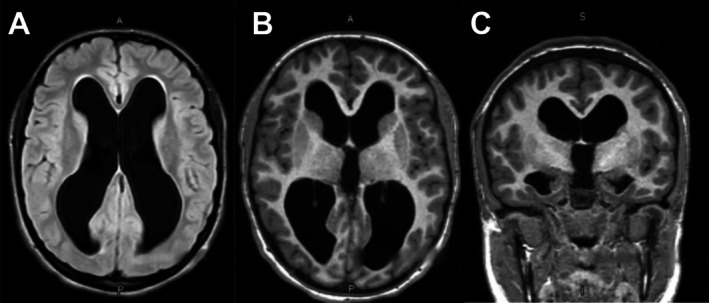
Preoperative axial (A and B) and coronal (C) MRI slices showing symmetrical dilation of the lateral and third ventricles with associated parenchymal thinning, consistent with noncommunicating hydrocephalus.
